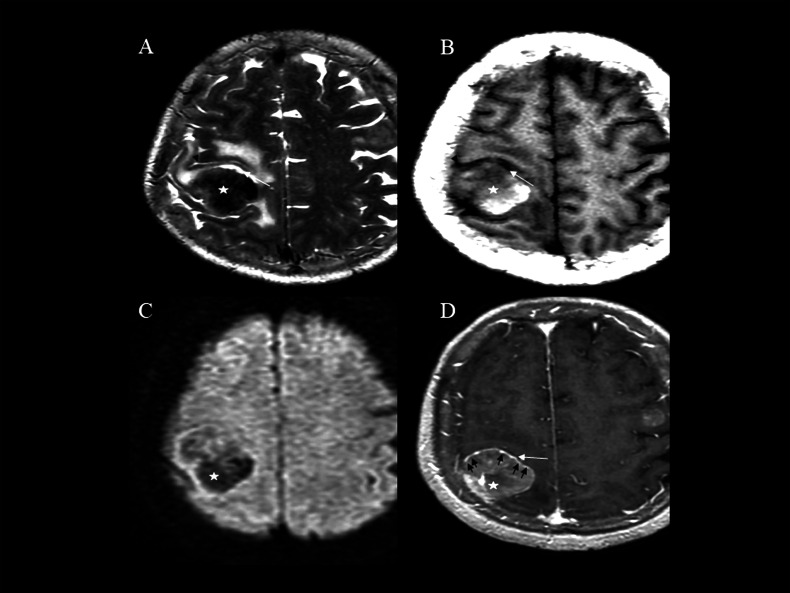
Representative case of the pool sign. (A): On T2WI, an arc-shaped region exhibiting higher signal intensity relative to the oedema band is observed between the tumor margin and the oedema band (white arrow). (B): On T1WI, the signal intensity of the corresponding area (white arrow) appears hypointense in comparison to gray matter. (C): On DWI, the region does not demonstrate diffusion restriction. (D): On contrast-enhanced T1WI, the region does not show enhancement (white arrow), although significant enhancement of the tumor's peripheral wall (black arrow) is evident. The black arrowhead indicates the cerebral sulcus between gray matter, and the star indicates the brain metastasis.
